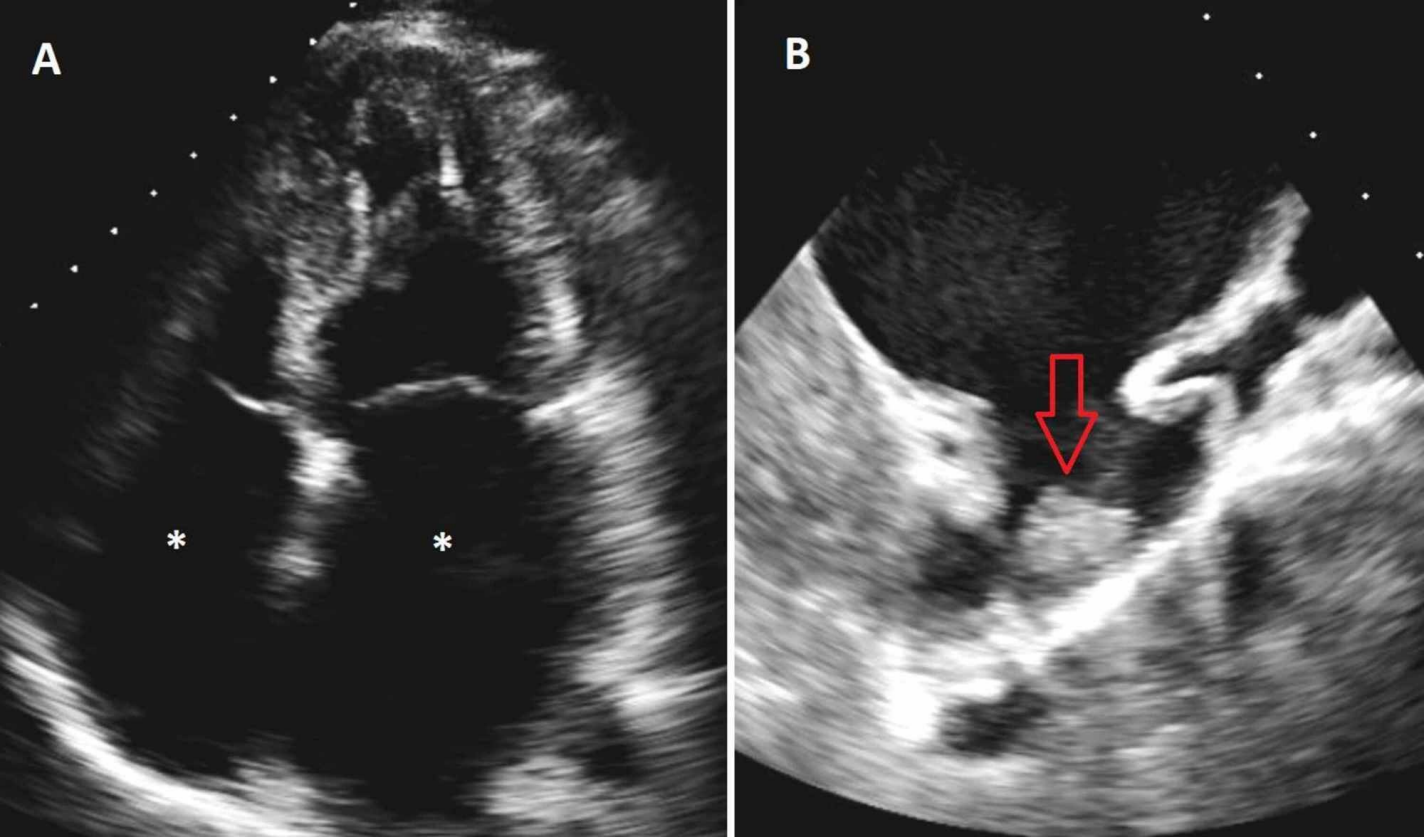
Transthoracic echocardiogram showing bi-atrial enlargement (white asterisks) (A), and transesophageal echocardiogram showing a left atrial appendage thrombus (red arrow) (B)
